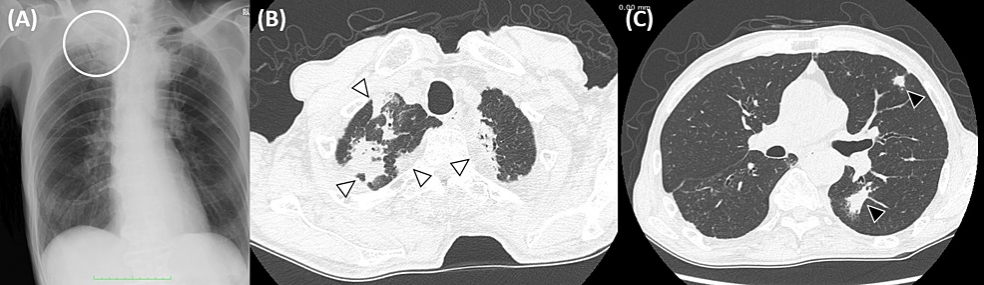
Chest radiographic and high-resolution CT images A) Chest radiography revealed an abnormal opacity of the right upper lobe lung (white circle). B, C) Axial high-resolution CT images of the chest revealed consolidations with cavitation in both upper lobes (white arrowheads) and solitary pulmonary nodules in the anterior segment and the left superior segment of the left lung (black arrowheads).
